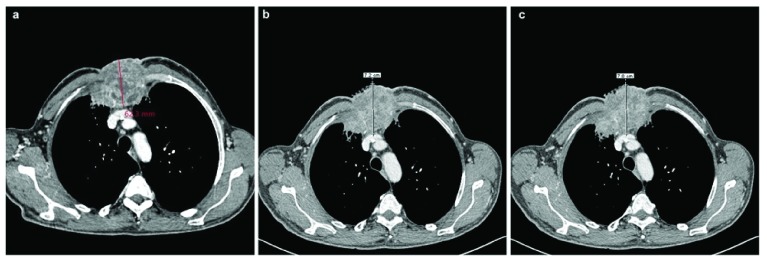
CT scan performed at baseline (
